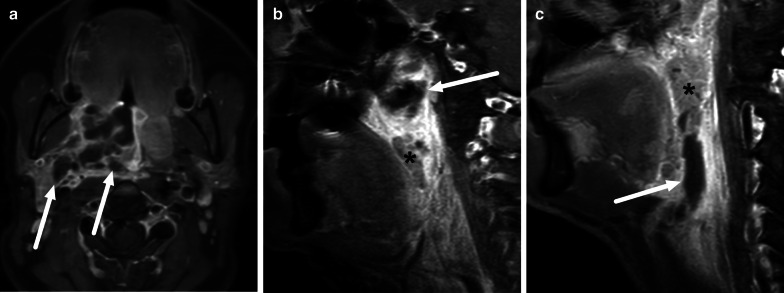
Examples of deep extension of PTAs on axial (a) and sagittal (b, c) fat-suppressed post-contrast T1-weighted images. Abscesses (arrows) can extend posterolaterally (a), superiorly (b), or inferiorly (c) from the tonsil (asterisk)
